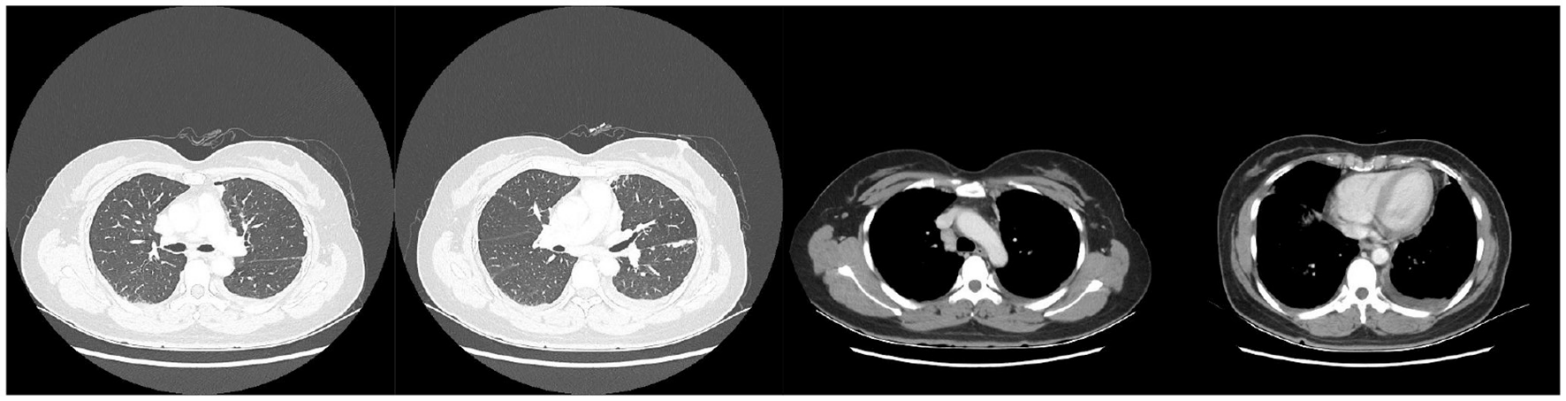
Chest CT showed pulmonary nodules, subpleural nodules, mediastinal lymphadenopathy and bilateral pleural effusion.
